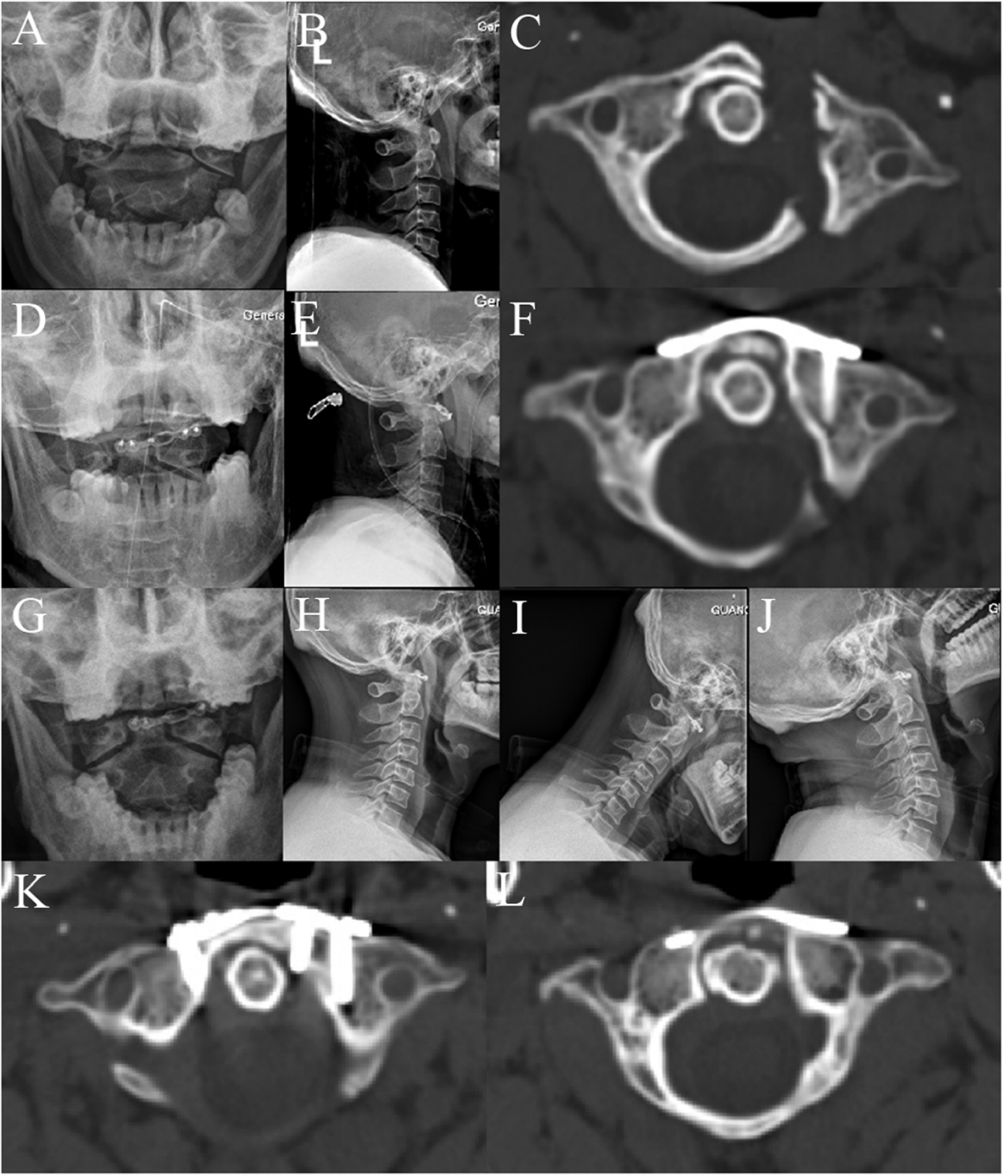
A 41-year-old female with atlas fracture treated by transoral anterior C1-ring osteosynthesis using a laminoplasty plate. Preoperative open-mouth (a) and cervical lateral X-rays (b), and axial CT (c) showed combined fracture of the anterior and posterior arches. Postoperative open-mouth (d) and cervical lateral X-rays (e) revealed the relatively good C1–C2 alignment. Axial CT (f) after surgery identified good reduction of the anterior and posterior arch fractures, and optimal placement of plate. Open-mouth (g), cervical lateral (h) and dynamic X-rays (i, j) at 9 months after surgery showed reliable fixation without instability of C1–C2. Axial CT images (k, l) at 9 months after surgery indicated satisfactory bone union of anterior and posterior arch fractures
